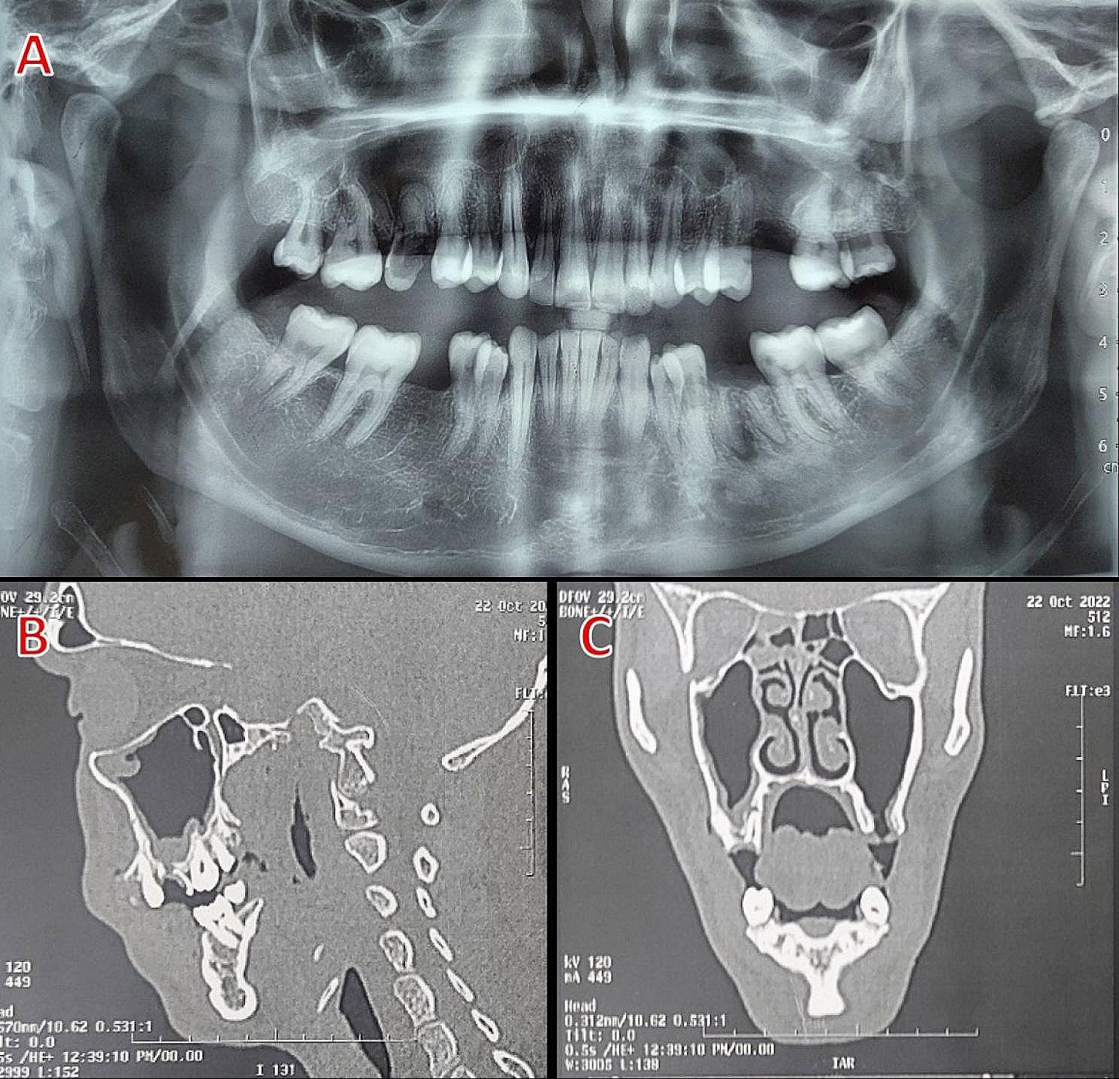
(A) Panoramic X-ray film demonstrating an oroantral fistula associated with the extracted upper left first molar. (B&C) A sagittal and coronal CT scan of the extraction site reveals an oroantral fistula, Case No. 2
